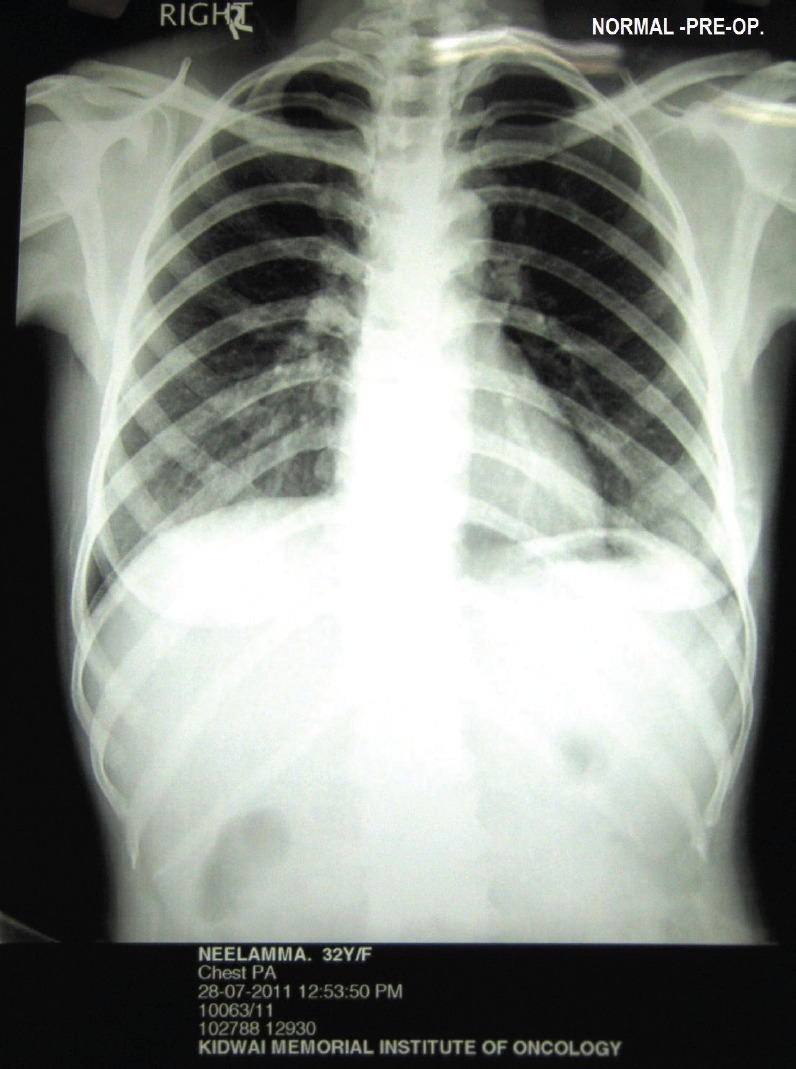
Normal pre-operative chest X-ray
